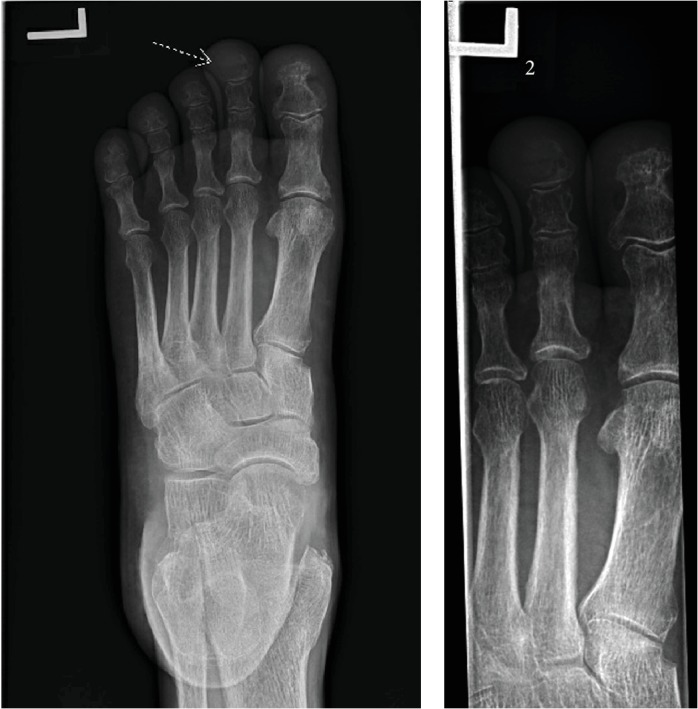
X-ray of left foot (AP lateral oblique views) demonstrating bony destructive lesion of the 2nd left toe.
